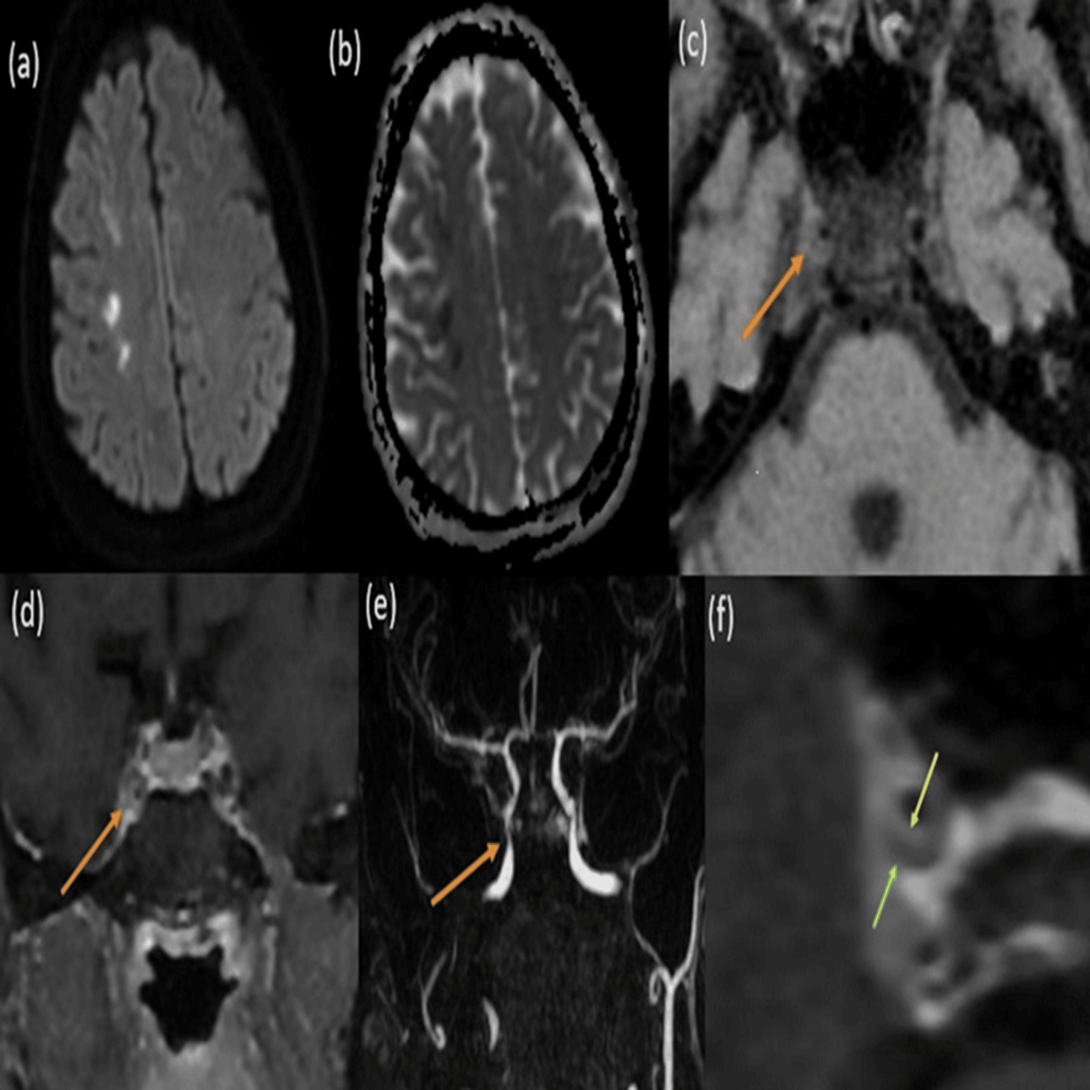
Intracranial atherosclerosis: (a and b) Axial DWI/ADC images demonstrating acute infarcts in the right subcortical and deep white matter of the right frontal lobe. (c and d) VW-MRI axial T1 BB and coronal T1 BB +C sequences demonstrating isointense eccentric wall thickening and grade 2 enhancement (orange arrows) involving a cavernous segment of the right ICA causing moderate stenosis demonstrated in CE-MRI sequence in (e) (orange arrow). (f) Magnified image of the site of a plaque showing a contrast-enhancing fibrous cap (yellow arrow) and non-enhancing plaque in the image (green arrow). DWI/ADC: diffusion-weighted imaging/apparent diffusion coefficient; VW-MRI: vessel wall magnetic resonance imaging; BB: black blood; ICA: internal carotid artery; CE-MRI: contrast-enhanced magnetic resonance imaging
